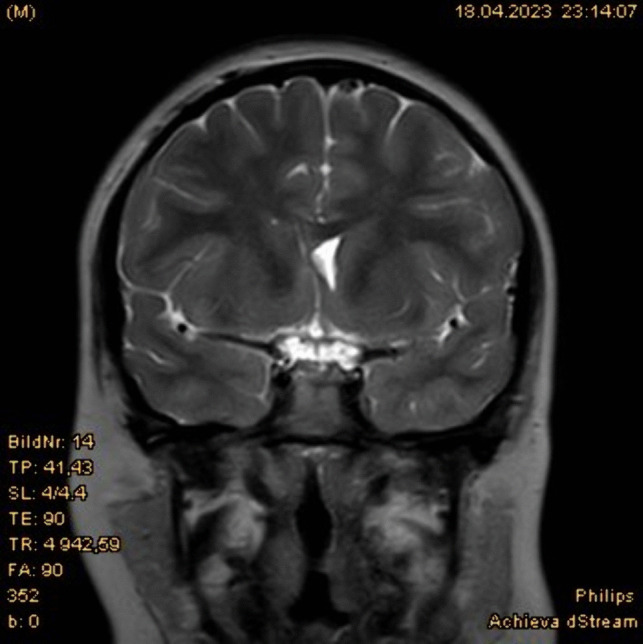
Postoperative MRI scan shows the tip of the catheter at the right frontal horn near the Foramen of Monroe and the reduced size of the right lateral ventricle
